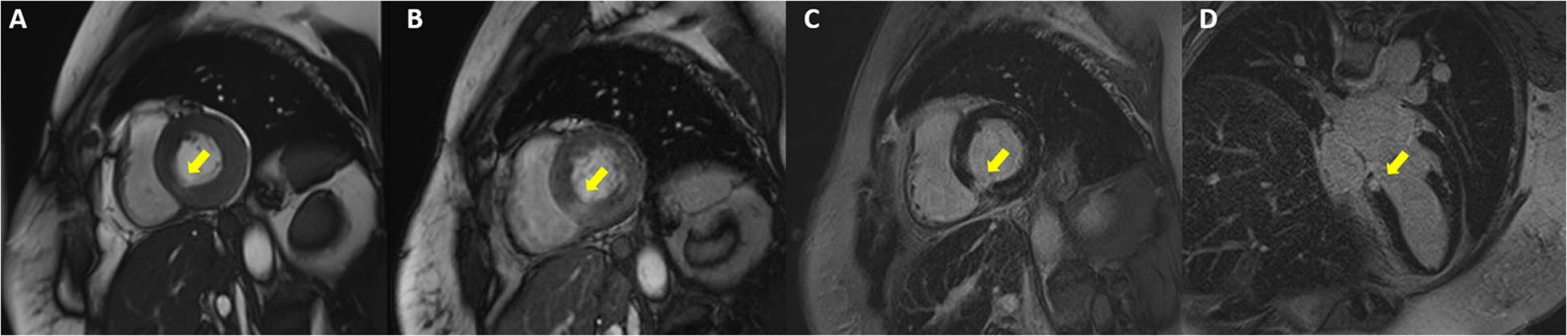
Cardiac magnetic resonance imaging with conventional techniques showed multiple signs of acute focal myocarditis at the basal inferoseptal segment of the left ventricle (arrows). A Steady-state free precession (SSFP) cine image in basal short-axis (SAX) view demonstrating regional wall motion abnormality. B Early gadolinium enhancement image in basal SAX view revealing hyperenhancement, a sign of myocardial hyperemia. C, D Delayed gadolinium enhancement images in basal SAX and modified four-chamber views depicting hyperenhancement, a sign of myocardial necrosis and fibrosis
